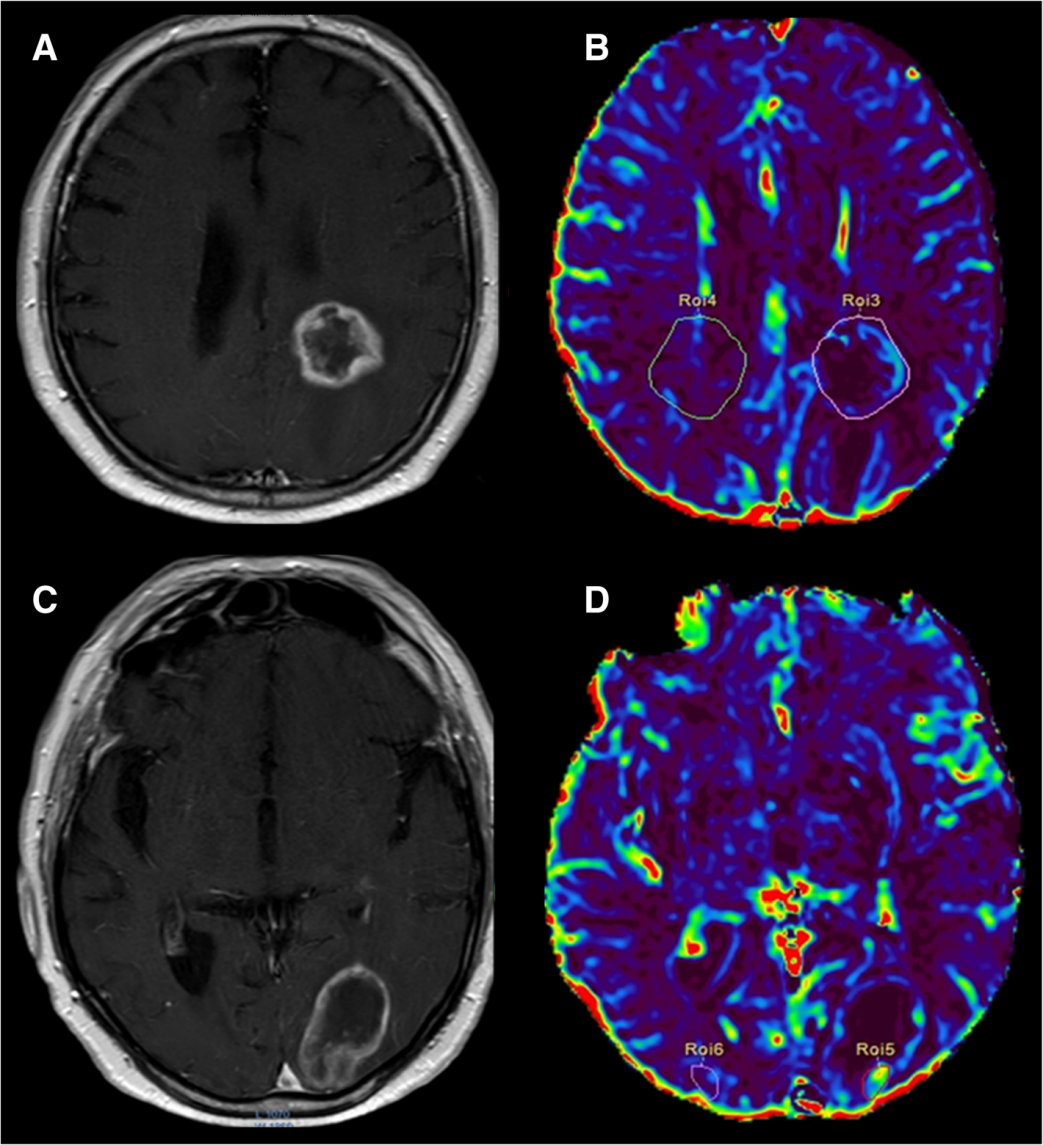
a-c (axial SE T1-weighted after Gadolinium administration), b-d (rCBV colorimetric map). Left paratrigonal and occipital brain metastases from lung cancer treated by SRS follow-up MRI examination. The enhancing left paratrigonal lesion shows low rCBV value according to radiation necrosis. The enhancing left occipital lesion shows a peripheral component with high rCBV value, according to tumor recurrence
